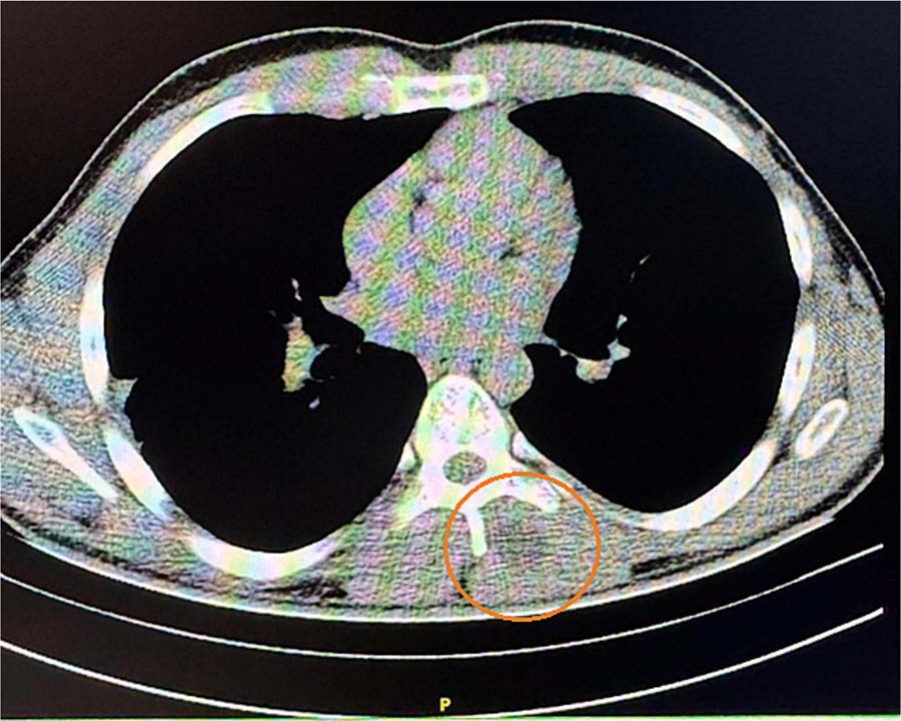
CT imaging of the formation preoperatively.
